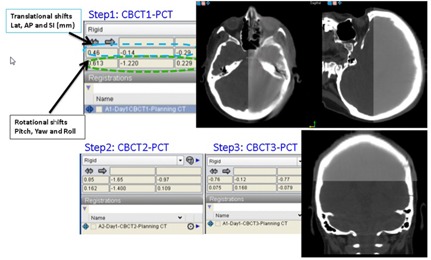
Representative clinical case: dark gray scale is the planning CT and the light gray is final the verification CBCT.
